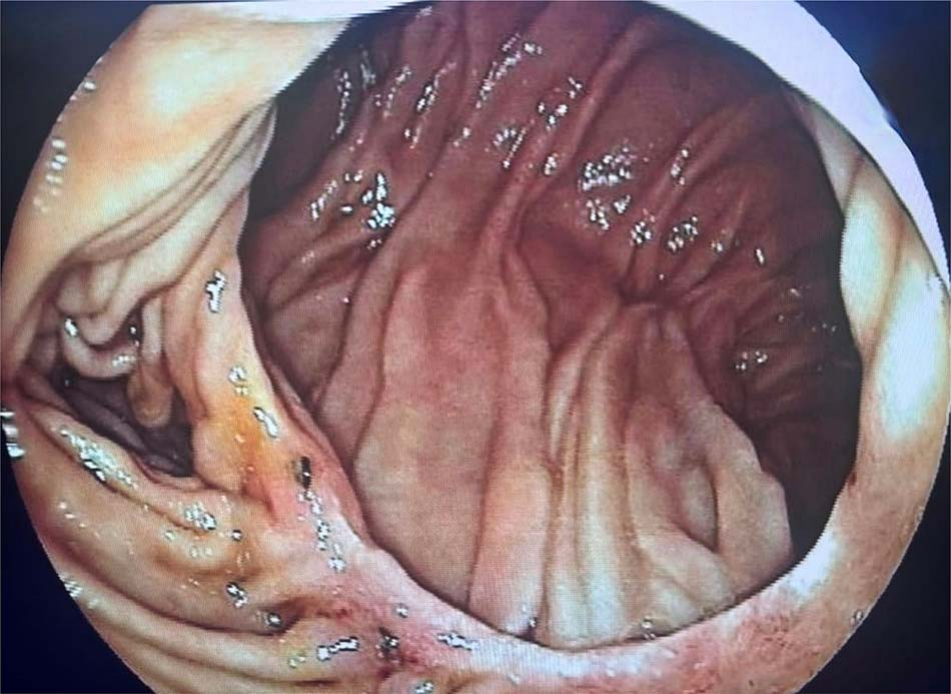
Contrast-enhanced abdominal CT demonstrating compression of the third portion of the duodenum: (A) axial view showing duodenal dilatation and abrupt narrowing; (B) sagittal reconstruction revealing a reduced aortomesenteric angle of 17°.
